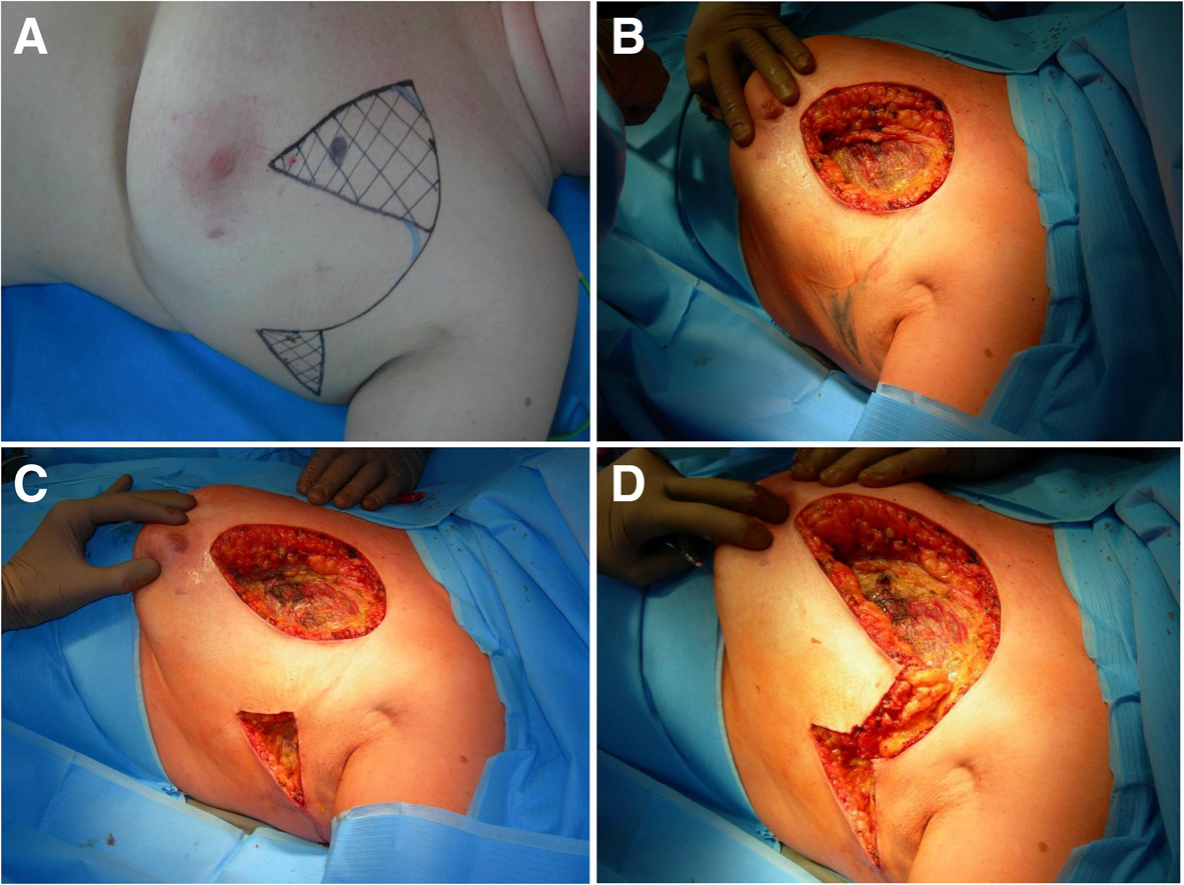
Breast segmentectomy, axillary approach, and connecting incision. a Skin markings. *Crossed lines*: triangles to be removed. Breast segment: extensive DCIS with invasive component. Hook-wire localisation needle inserted into invasive mass (*black oval*) under ultrasound guidance. b Breast segmentectomy: triangular full-thickness excision in a radial fashion. c Excision of axillary triangle (skin and fat tissue) at the superior area of axilla. d Incision connecting the bases of triangles (*upper quadrants*). Easy approach to the axillary lymph nodes
